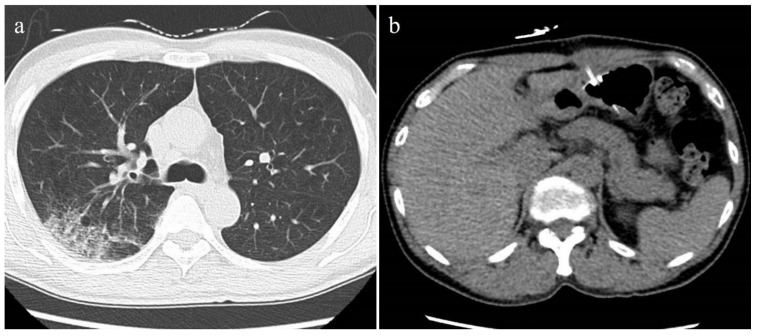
Unenhanced axial CT images of a 57-year-old male patient with non-severe COVID-19 pneumonia. (a) Chest CT showed the ill-defined ground-glass opacity in the middle lobe of the right lung. The lung severity score was 0.4. (b) No abnormal findings were observed in the pancreas and liver on the abdominal CT. The CT-FVF of the pancreas was 4.5 % and the CT attenuation value of the liver was 61.2 HU.
